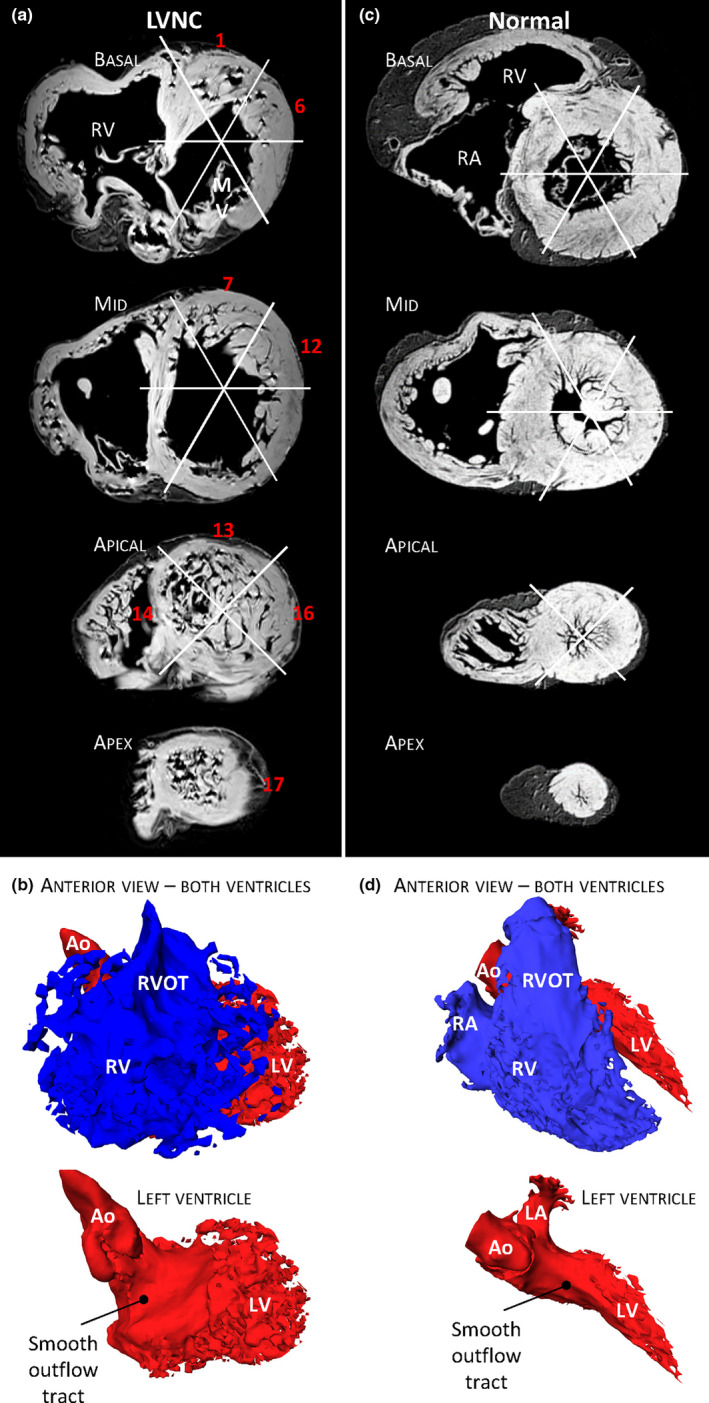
Excessive trabeculation Case 1 compared with a normal heart. (a) MRI images of Case 1 from which all 17 segments were assessed. Numbers in red indicate the segment number with a T/C greater than 2.3 (excessively trabeculated (Petersen et al., 2005)). (b) Ventricular lumens cast in anterior view, showing a spongy appearance to the left ventricular lumen (LV) apically, laterally and basally. (c) MRI images of a normal heart, no segments had a T/C greater than 2.3. (d) Ventricular lumens cast in anterior view, showing a less spongy left ventricular lumen than in (b). Ao, aorta; LA, left atrium; Mv, mitral valve; RA, right atrium; RV, right ventricle; RVOT, right ventricular outflow tract [Colour figure can be viewed at wileyonlinelibrary.com]
